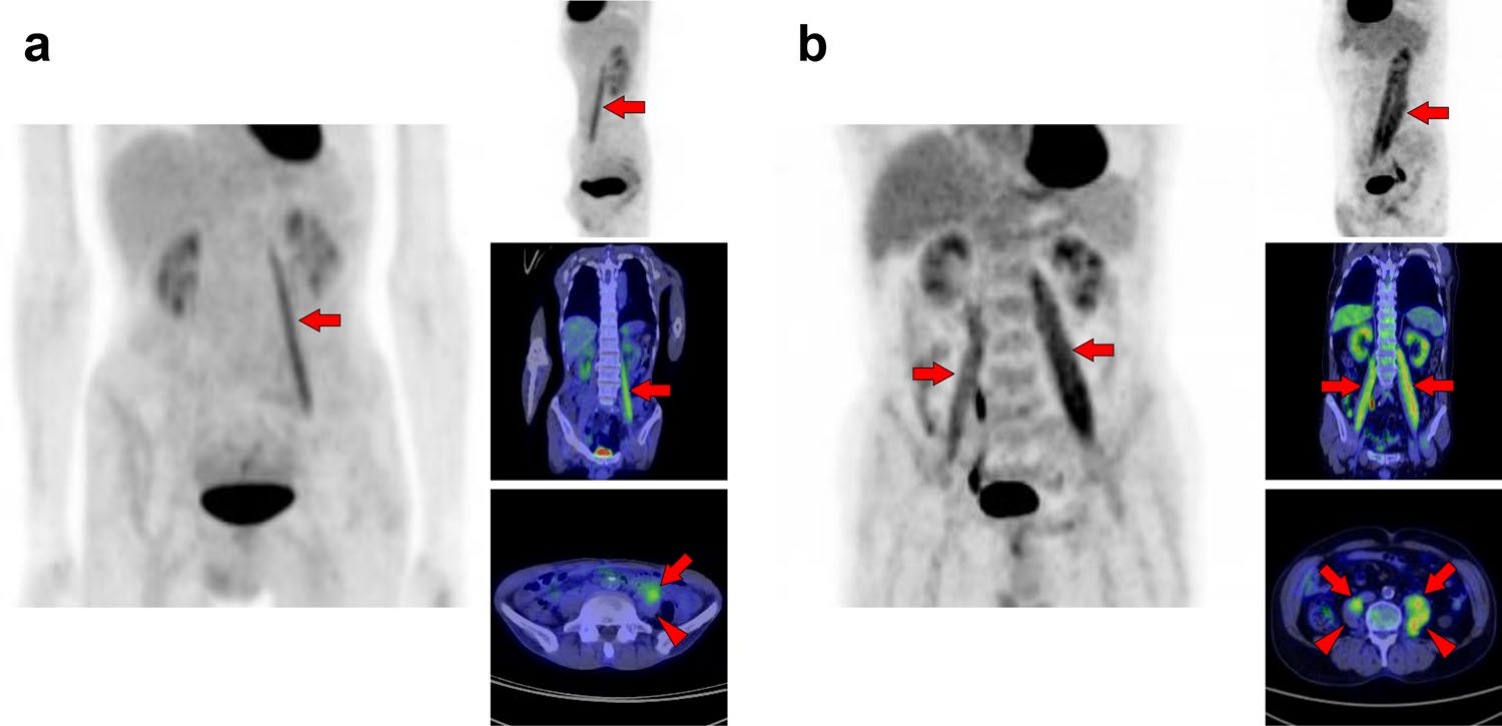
Psoas major muscle.* [^18^F]FDG uptake in the psoas major is often visualized bilaterally but can also be unilateral or have strong laterality (a, b). The psoas major has superficial (arrows) and deep (arrowheads) parts, and uptake in the superficial tends to be dominant
